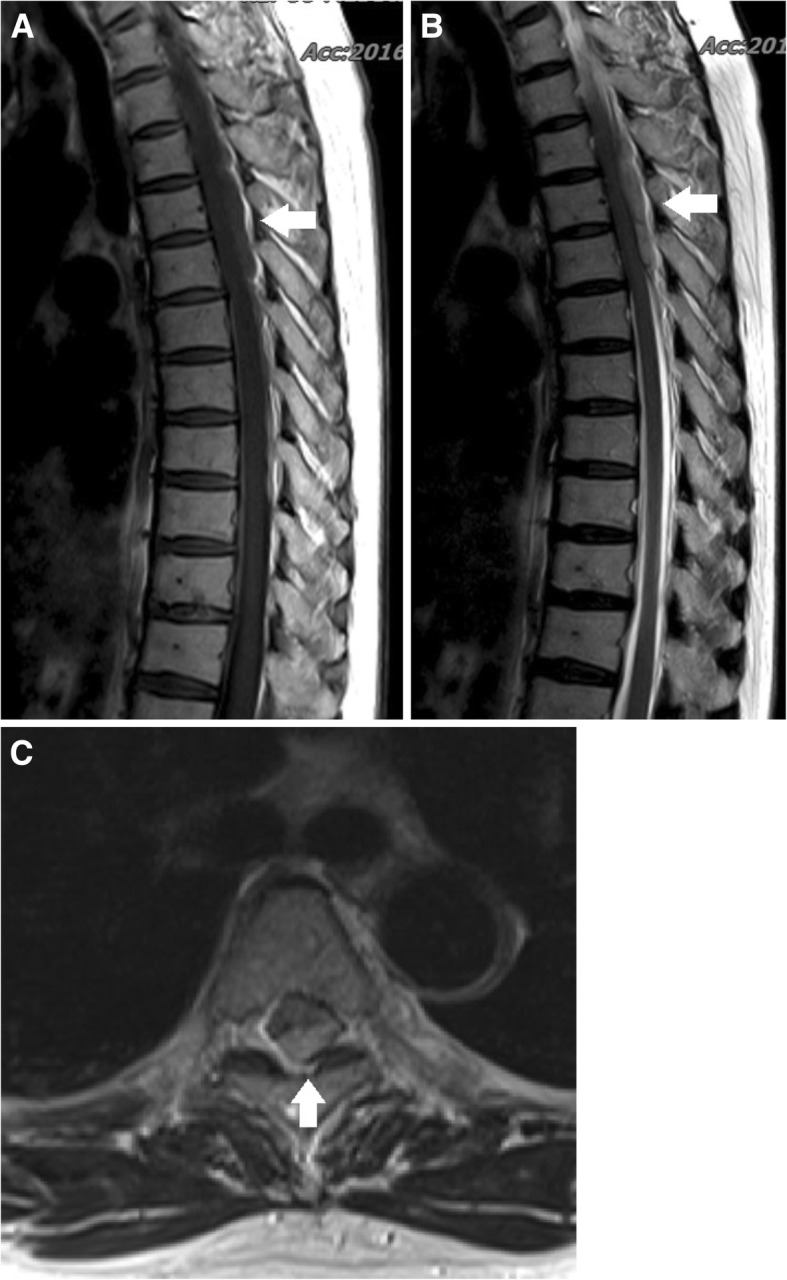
(a) Preoperative T1-weighted MRI shows iso signal intensity posterior spinal epidural mass lesion (sagittal image) (b) Preoperative T2-weighted MRI shows high signal intensity posterior spinal epidural mass lesion(sagittal image). (c) Axial image shows epidural hematoma on the dorsal portion of spinal canal (T2-weighted MRI)
